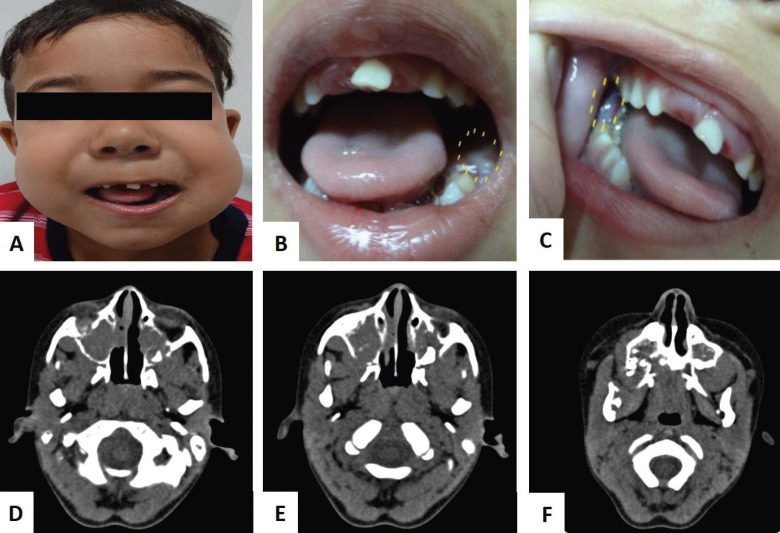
(A-C) Initial clinical facial asymmetry. Frontal view of the face prior to the intraoral incisional biopsy. Intra-oral maxillary jaws swelling causing limited mouth opening and ulceration in the left alveolar ridge. (D-F) Axial CT scan showing mass invasion causing destruction of orbits floor, both maxillary sinus and maxilla.
